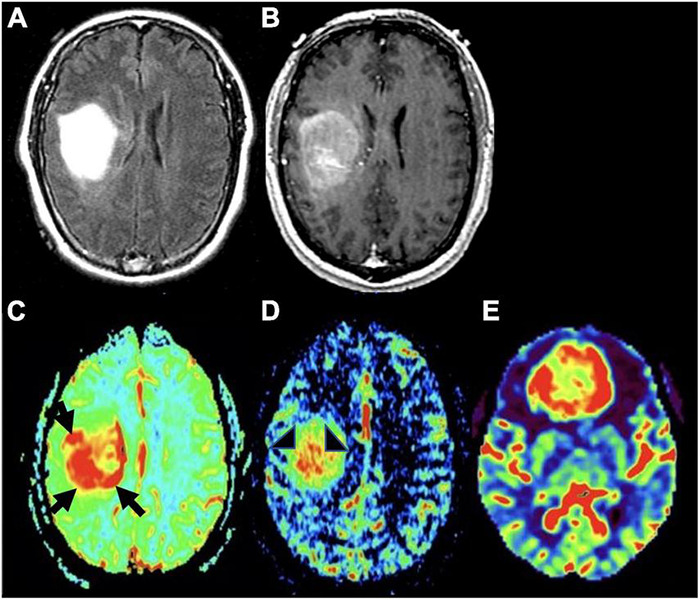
Molecular glioblastoma. (A) Axial FLAIR: homogeneously hyperintense mass; (B) axial T1 post-contrast: mild enhancement within the mass without distinct area of necrosis; (C) axial DSC perfusion: marked hyperperfusion within the lateral and posterior aspects of the mass (black arrows); (D) axial DCE perfusion: marked capillary leakiness within the central aspect of the mass (arrowheads); (E) axial ASL perfusion: marked increase in cerebral blood flow and hyperperfusion of tumor in the frontal lobe (different tumor than the one depicted in panels A–D).
